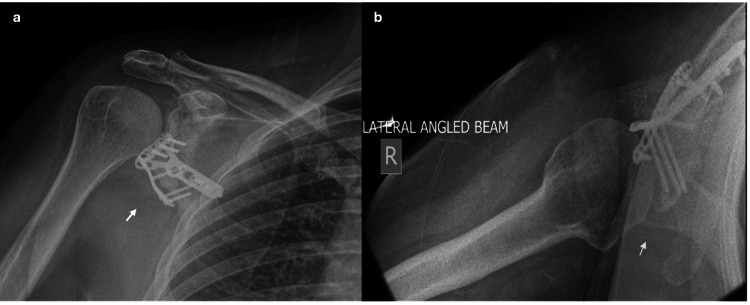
Six-week post-operative radiographs of the right shoulder. a. Anterior-posterior right shoulder X-ray. b. Axial right shoulder radiograph. Figures 4a-4b show a well-maintained reduction and stable position of the distal radius plate six weeks post-operatively (as indicated by arrows).
